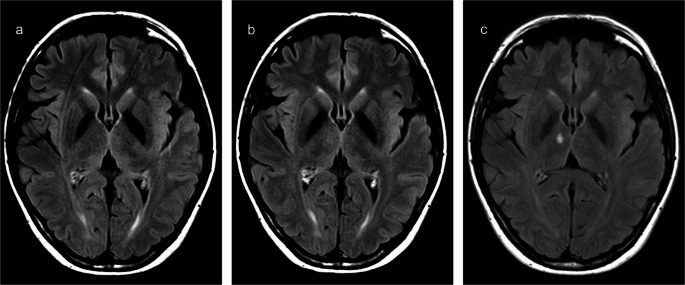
FLAIR signal changes during the hyperacute (a), acute (b), and chronic (c) stages of the right ventromedial thalamic stroke
